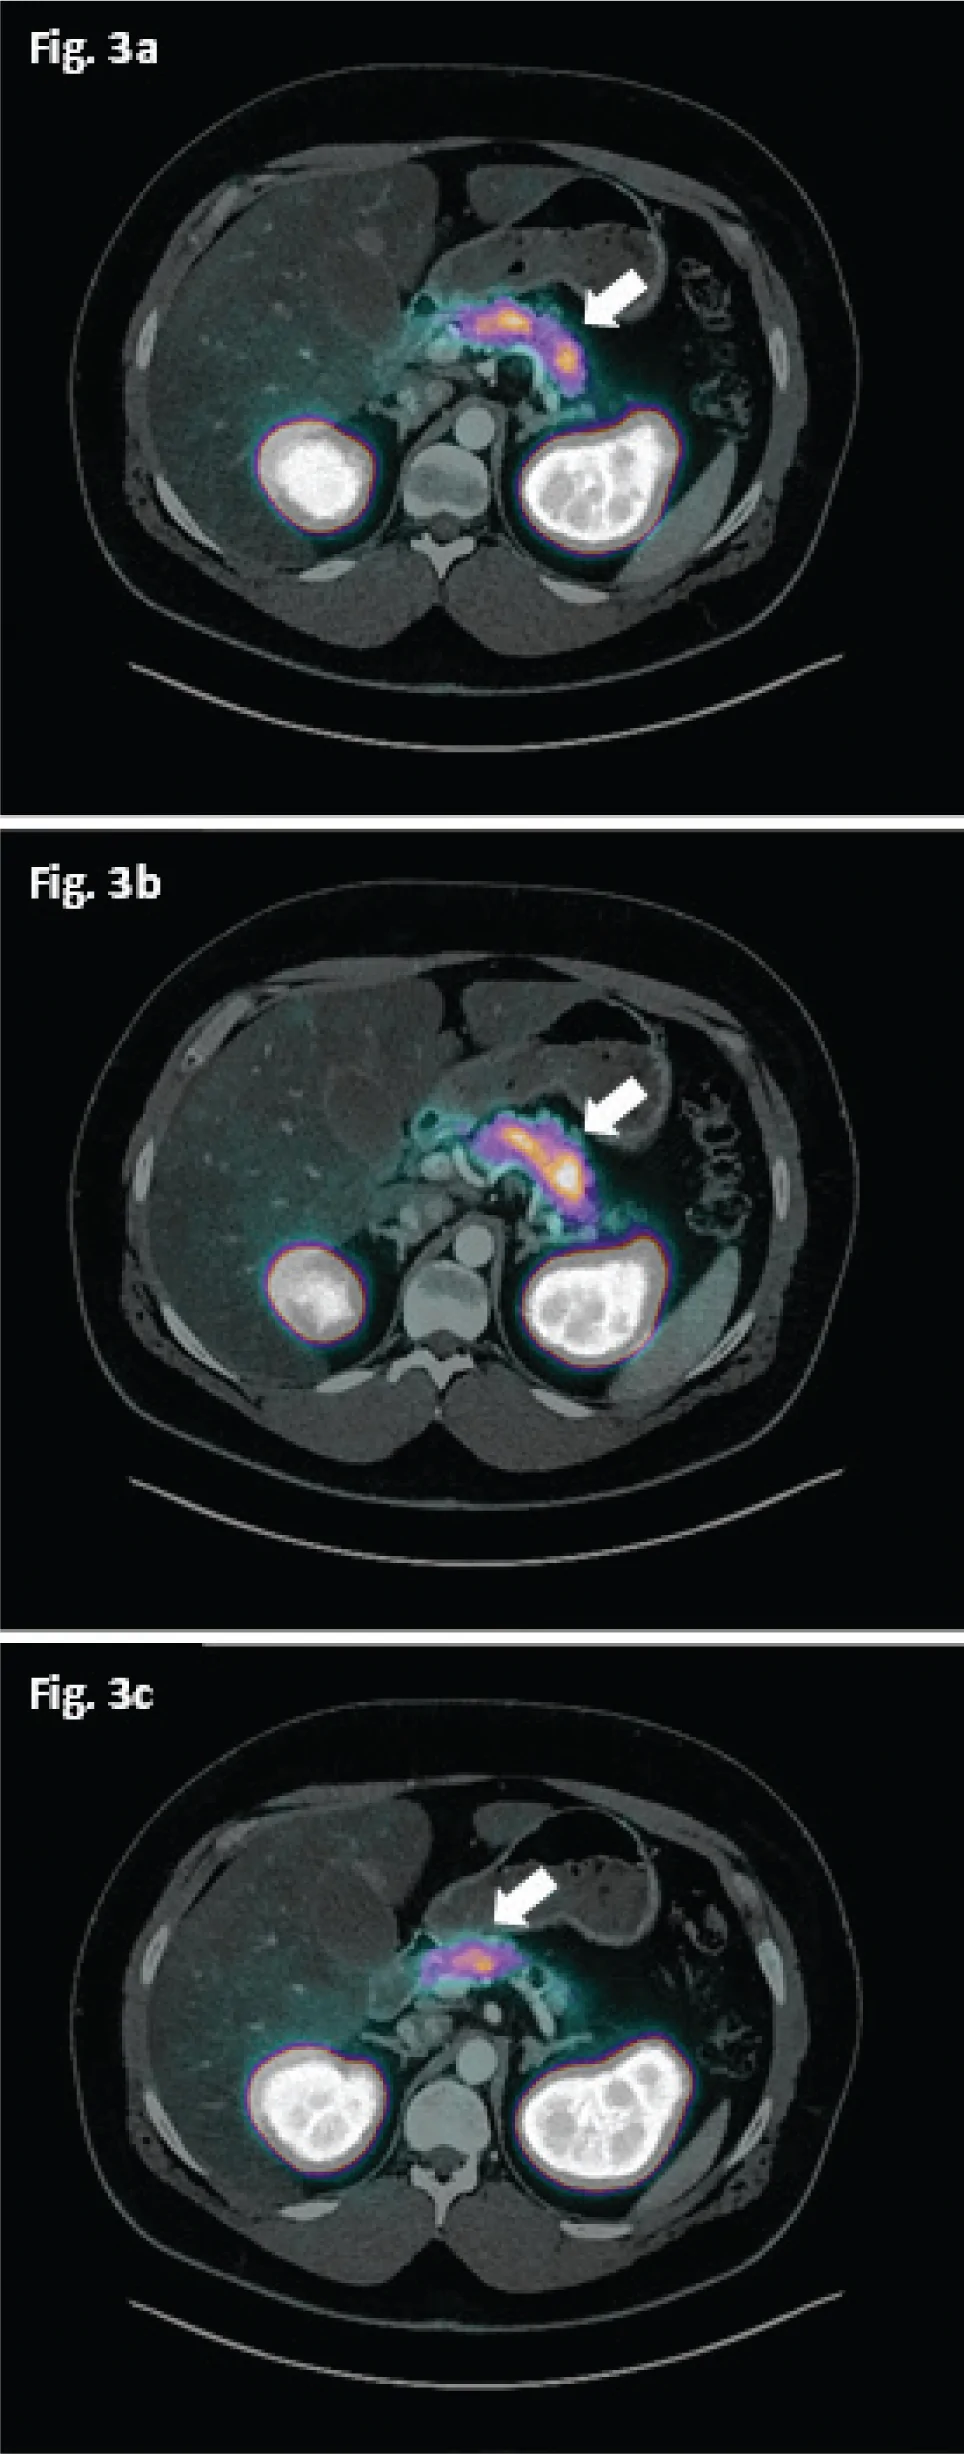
^68^Ga-NOTA-exendin-4 PET/CT images showing GLP-1 receptor uptake in the pancreatic region in the context of MEN1-associated multifocal pancreatic neuroendocrine tumors. Consecutive axial fused PET/CT images demonstrate uptake corresponding to the pancreatic lesions, indicated by arrows. No single dominant GLP-1R-avid lesion was identified to account for the patient’s hyperinsulinemic hypoglycemia, limiting surgical localization. PET/CT: positron emission tomography/computed tomography; GLP-1: glucagon-like peptide-1; MEN1: Multiple Endocrine Neoplasia type 1.
